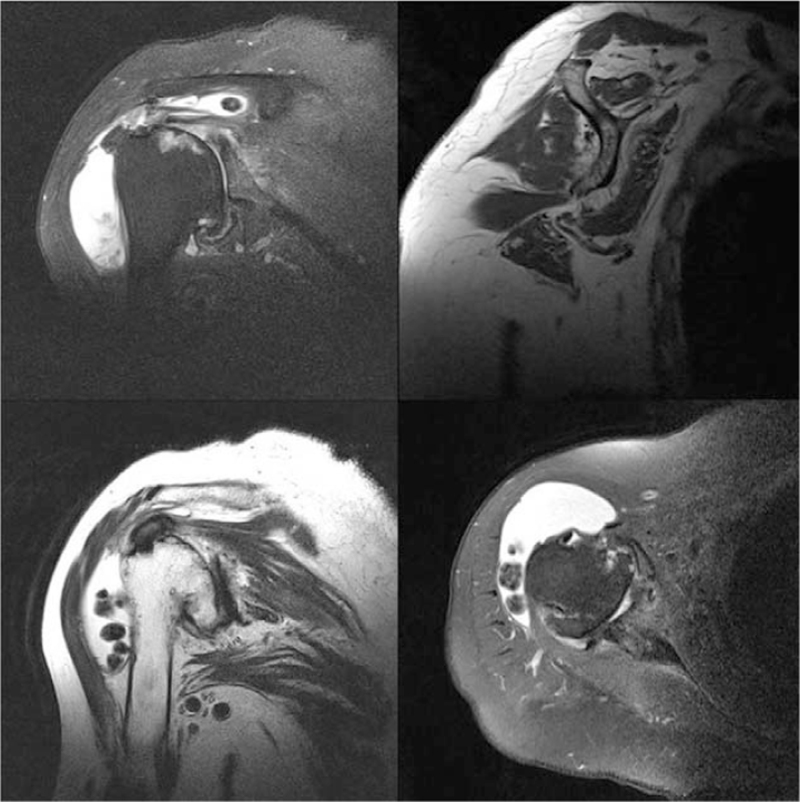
Magnetic resonance imaging demonstrating a full-thickness tear and retraction of the supraspinatus and partial infraspinatus tendon with diffuse atrophic changes in the muscle. Large amounts of fluid and multiple similarly sized loose bodies are seen in the subacromial subdeltoid bursa. Hypertrophy of the synovium is also seen.
